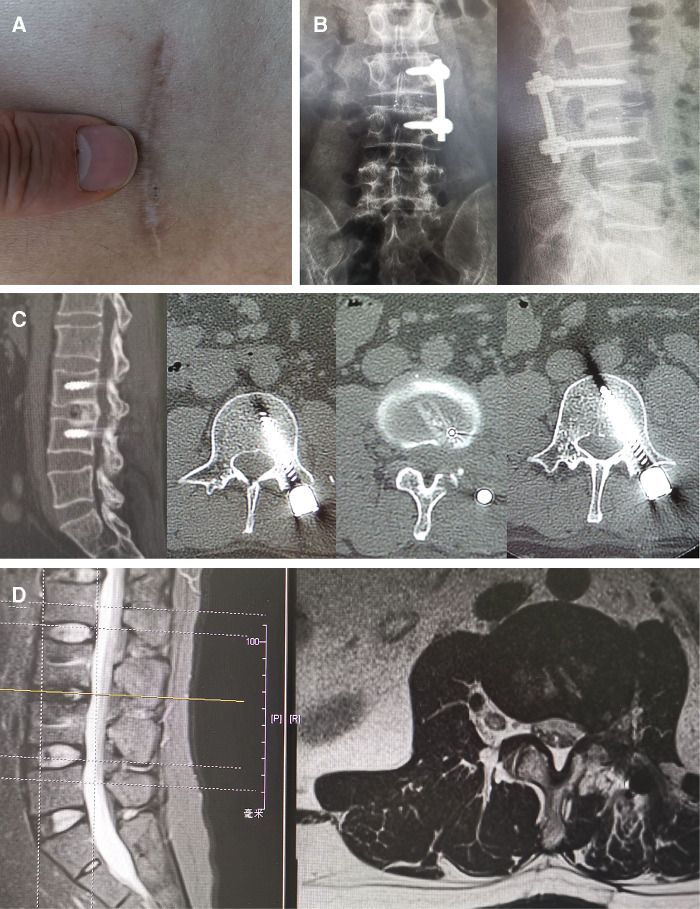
X-rays and CT scans showed the fusion of intervertebral segments was achieved, and there was no failure of internal fixation and occurrence of spinal deformity at 5 years after surgery. Grade I fusion was attained in a lumbar dumbbell-shaped tumor patient at 5-year follow-up. There was no recurrence of any spinal tumor 5 years after surgery confirmed by MRI examination. (A) The minimally invasive incision was shown 5 years after surgery. (B) x-rays; (C) CT scans. (D) MRI of lumbar dumbbell-shaped tumor patient (case 5).
